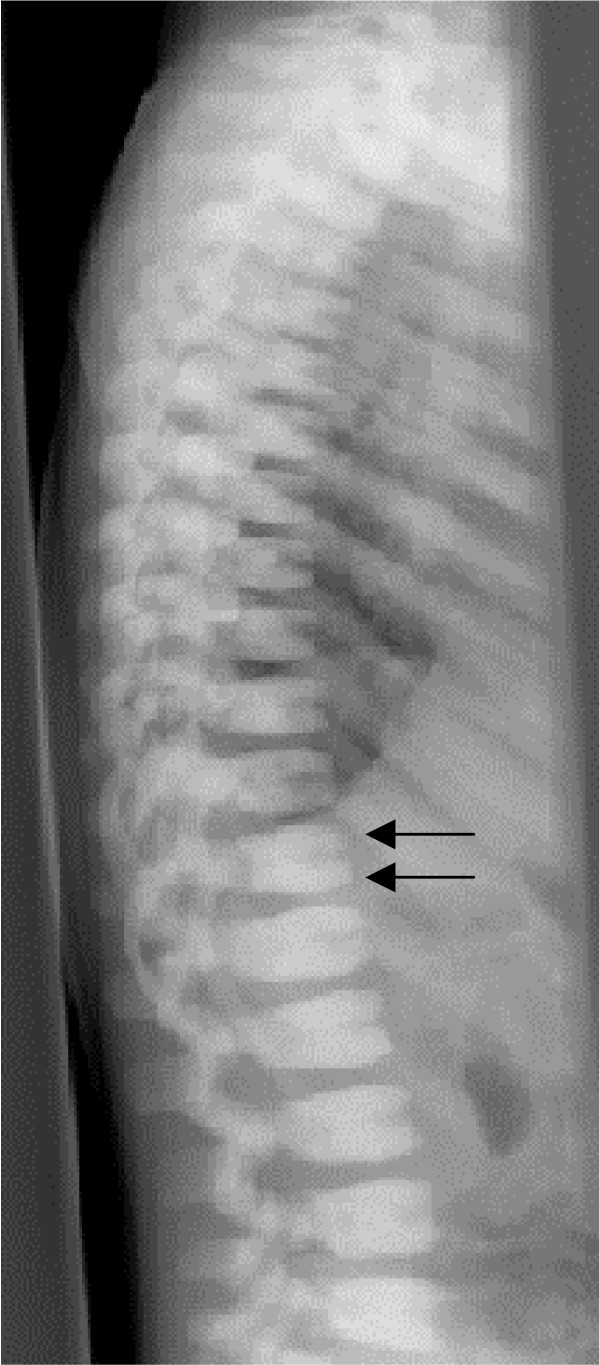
ADO: lateral spine radiograph, age 4 years. Note sclerosis of vertebral endplates (arrows) resulting in 'sandwich vertebrae' appearance.
